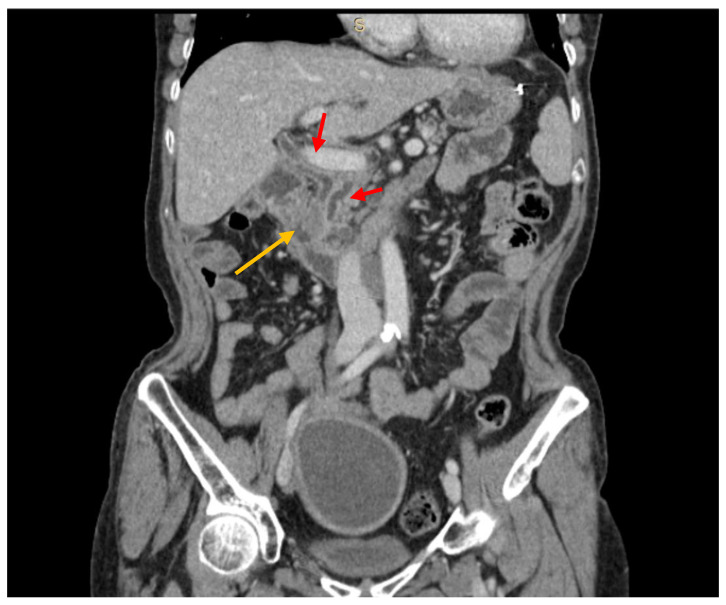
CT scan showing a hypovascular lesion (yellow arrow) of the pancreatic head, causing both bile duct and panceatic duct dilation (red arrows) (PM from colon cancer).
